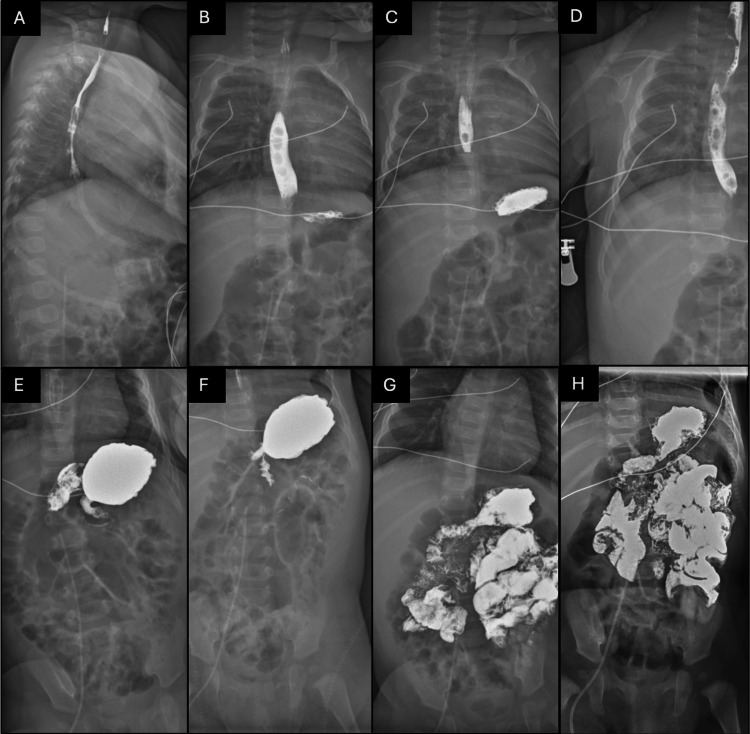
X-ray upper GI series after contrast ingestion with small bowel follow-through GI: gastrointestinal (A) Lateral view showing initial contrast passage through the esophagus. (B) Anteroposterior view demonstrating contrast within the mid-esophagus. (C) Continued esophageal transit with contrast entering the stomach. (D) Contrast outlining the distal esophagus without evidence of obstruction. (E) Contrast within the stomach with the normal configuration. (F) Gastric emptying with passage of contrast into the duodenum. (G) Contrast progression through proximal small bowel loops. (H) Delayed image demonstrating normal distribution of contrast throughout the small intestine without evidence of malrotation or obstruction
